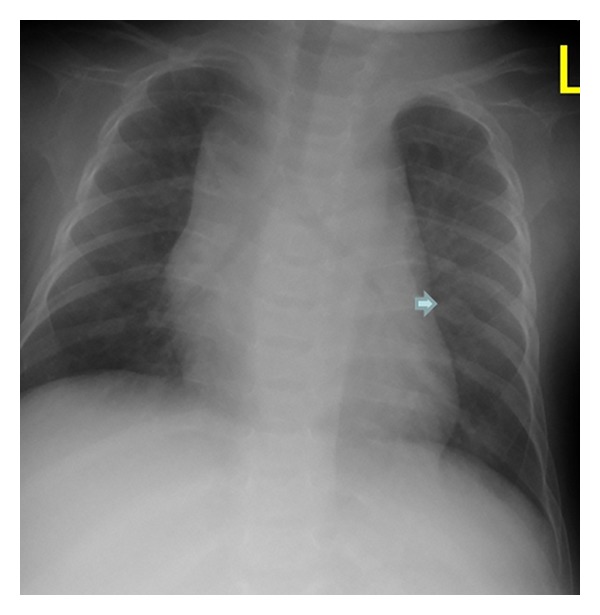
Anteroposterior thorax radiograph generalized osteoporosis associated with thin and somehow gracile and short ribs. Note callus formation on the 7th right rib (arrow).
